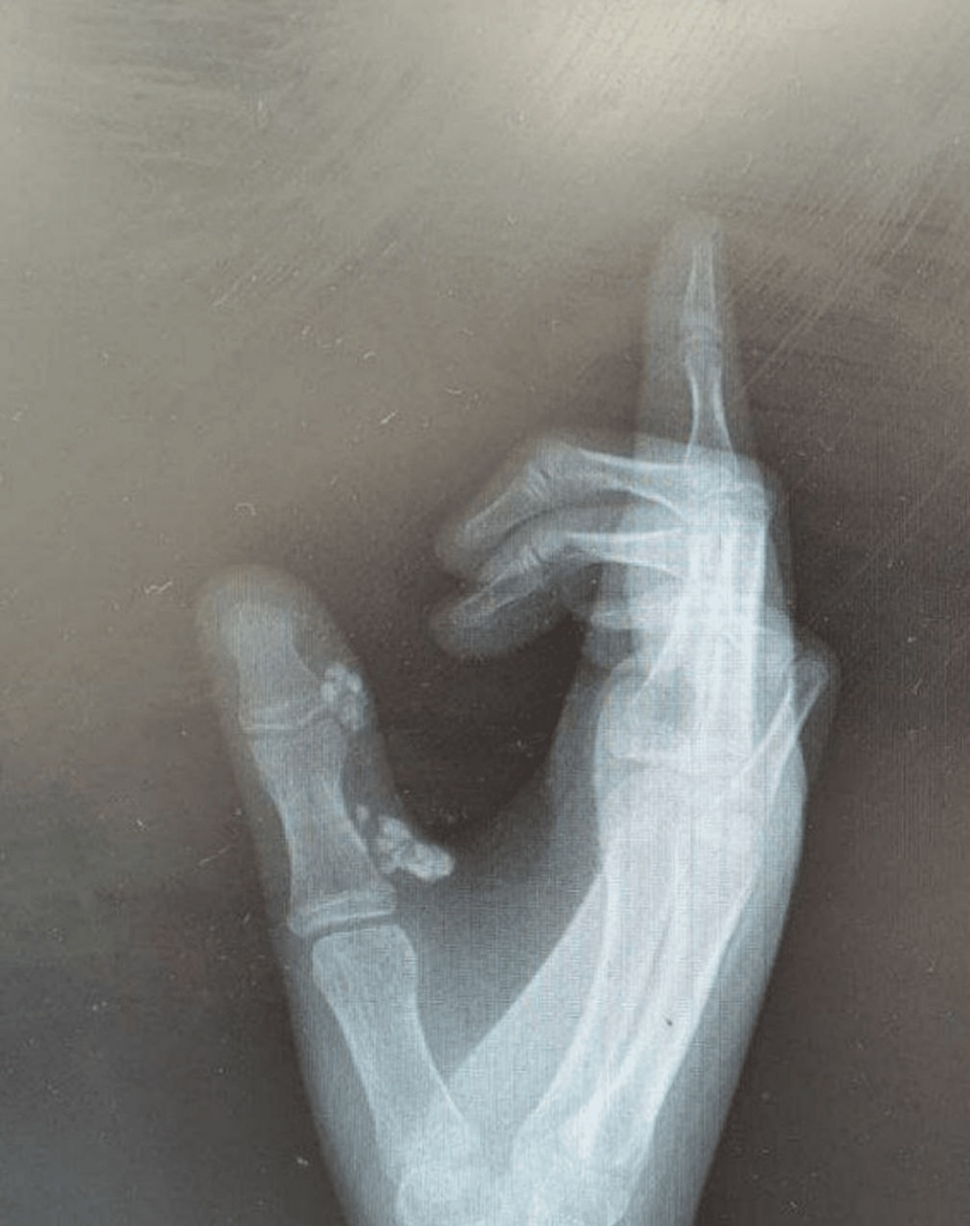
Plain X-ray of the calcinosis cutis specifically affecting her right thumb's interphalangeal joint and metacarpophalangeal joint
